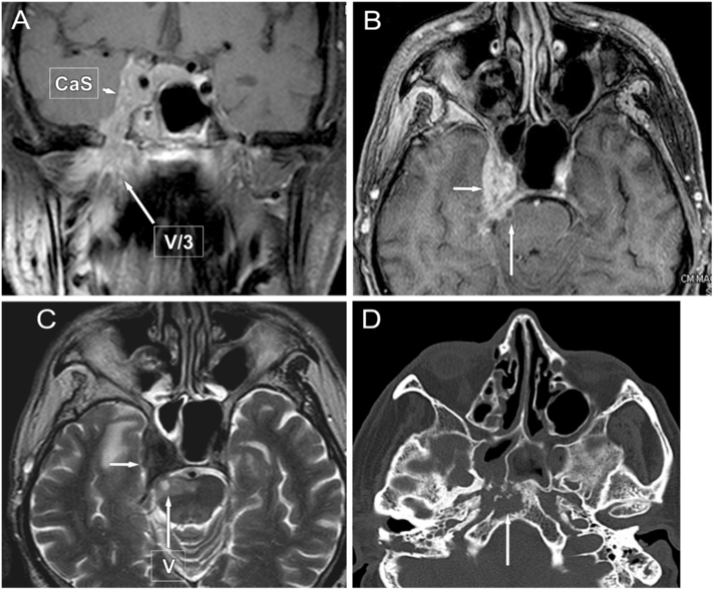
Extensive nasopharyngeal cancer with skull base destruction and perineural spread into the brainstem. (T4). A, B: coronal and axial post-contrast T1-weighted MR image with fat suppression. A image shows tumor extension from the nasopharynx through the right wide foramen ovale and perineural spread along the V/3 (long arrow) into the cavernous sinus (CaS, arrow-head). The CaS is flared out towards the Meckel’s cavity and tumorous enlargement of the trigeminal ganglion (semilunar, or Gasser) can be well seen (on B, C images, short arrows). C: axial T2-weighted image shows signal intensity changes in the pons secondary to the perineural tumour spread along trigeminal nerve root (on B, C images, long arrows) D: axial nonenhanced CT scans show tumorous bone destruction of the clivus on the right side (long arrow).
